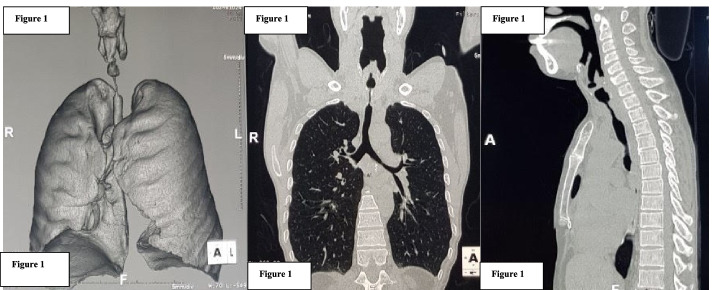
CT scan image of tracheal stenosis after intubation
